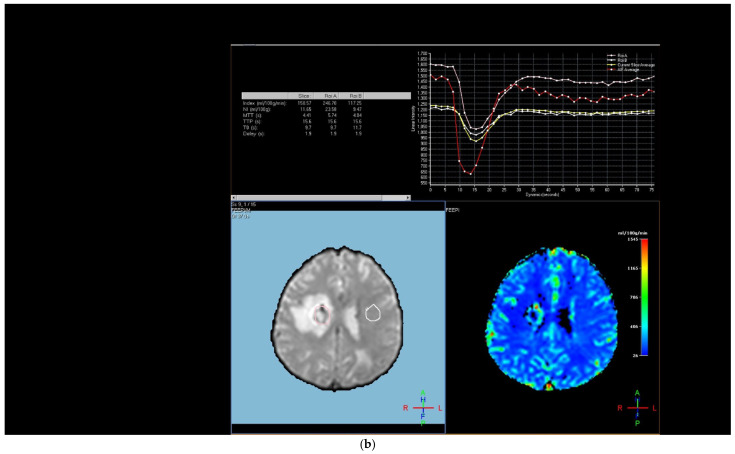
Brain magnetic resonance imaging (MRI) of a 67-year-old male with small-cell lung carcinoma revealed (a) peripherally enhanced right periventricular mass on post-contrast axial T1-weighted image and (b) hyperperfusion with a rCBV of 2.4 in perfusion-weighted imaging. Pink ROI is from metastatic nodule and white ROI is from contralateral parenchyma.
